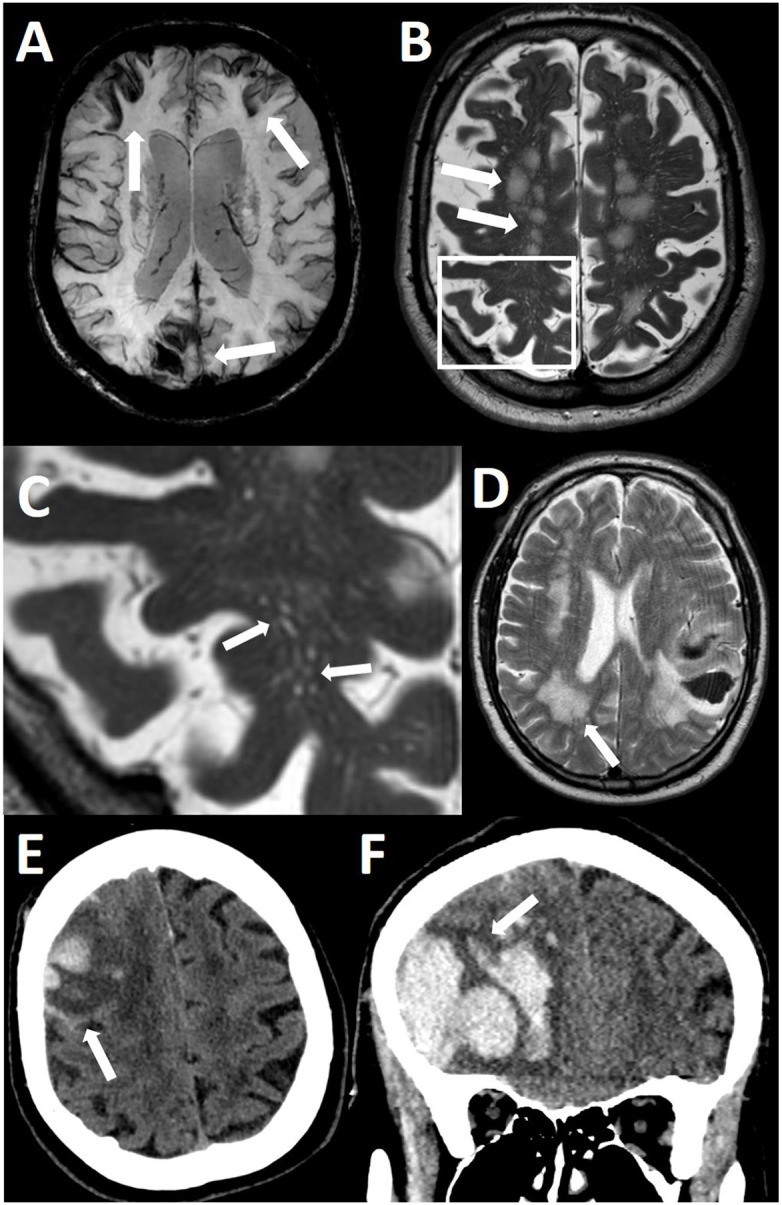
MRI and CT features in cerebral amyloid angiopathy. (A) Cortical superficial siderosis (arrows) on an axial susceptibility-weighted image of the brain. (B) Subcortical white matter lesions (arrows) on a T2-weighted axial image of the brain. (C) Inlet magnification of B, MRI-visible perivascular spaces in the centrum semiovale. (D) Dorsal white matter caps (>5 mm, arrow). Note the left parietal lobar hemorrhage. (E) Subarachnoid hemorrhage (arrow) adjacent to the main hemorrhage. (F) Finger-like projection exiting the main hemorrhage as an extension longer than its width at the exit from the main hemorrhage (arrow).
